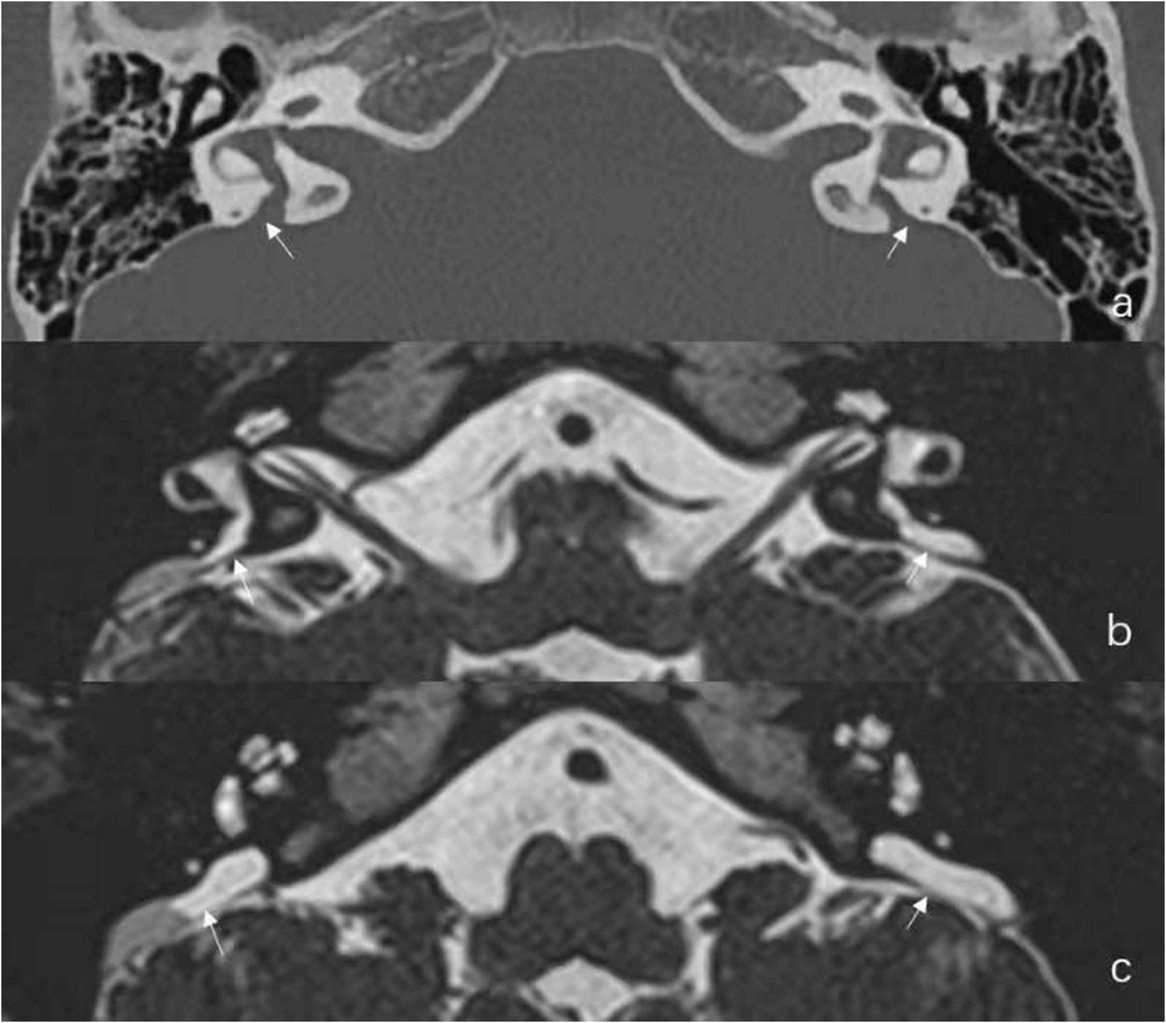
Radiological results of Case No. 2. (a) Axial temporal bone CT reveals the entire course of the large vestibular aqueduct extending from the crus communis of the vestibule to the posterior aspect of the petrous bone. (b,c) Additional axial Three-dimensional sampling perfection with application optimized contrasts using different flip angle evolutions (3D-SPACE) Magnetic Resonance Imaging (MRI) in the same child reveals enlarged endolymphatic duct (ED) and large endolymphatic sac (ES) containing variable signal intensity.
